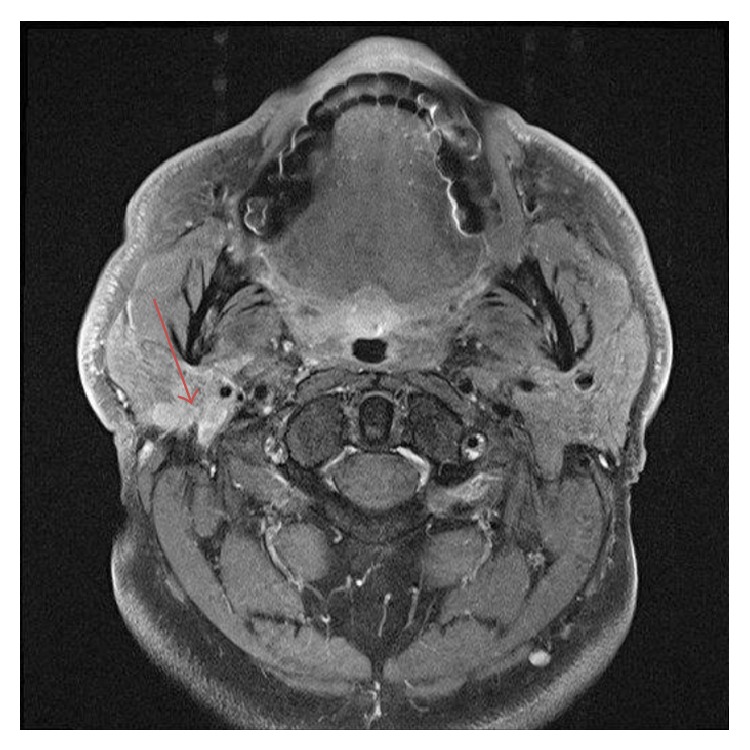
MRI of the neck, axial section (T1-weighted), showing a mass in the deep lobe of the right parotid gland (arrow).
